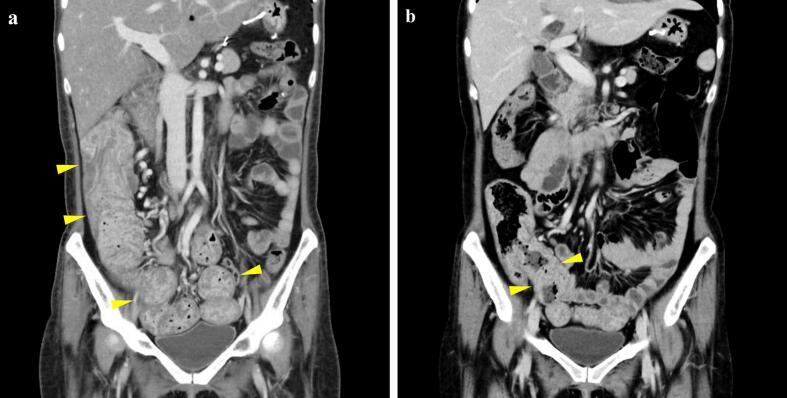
Abdominal computed tomography images (a) 21 days and (b) 121 days after initiation of infliximab. Multiple mass shadows suspected to be ileal and colonic polyps (arrowheads) show reduction in size.
